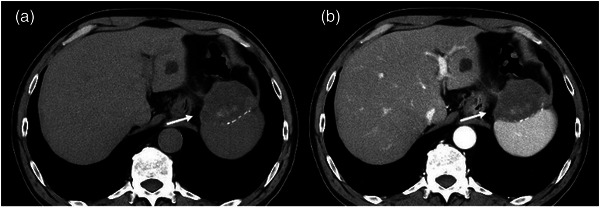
Plain computed tomography (a) showing calcification at the margins and inside of the mass. No clear contrast enhancement effect was observed on contrast‐enhanced computed tomography (b).
